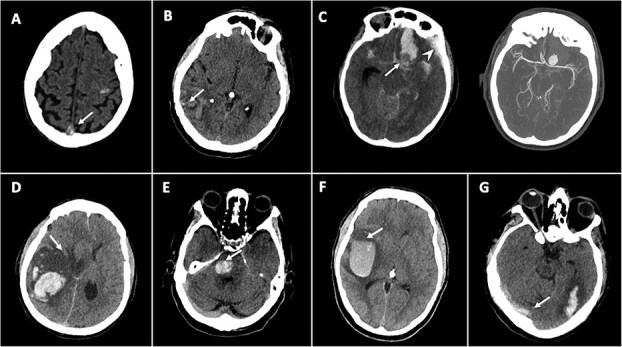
Examples of intra-parenchymal haemorrhagic NCCT findings suggestive of secondary causes of ICH. (A) Small, concave-shaped haemorrhage in the left frontal Rolandic juxtacortical white matter (“cashew nut sign)” with spontaneous hyperdensity of the superior sagittal sinus (arrowhead) suggestive of cerebral venous thrombosis. (B) Right parietal haemorrhage with calcifications (arrow) suggestive of an arteriovenous malformation. (C) NCCT shows a “flame” shape haemorrhage in the left paramedian frontal lobe near a round hypodensity—visible as a concave, “minus” in the haemorrhage (arrow) with associated subdural haemorrhage (arrowhead). Multidetector CTA shows a large saccular aneurysm of the left middle cerebral artery. (D) Large, irregular ICH with a disproportionate perihaematomal oedema (arrow), suggestive of an underlying tumour. (E) Round hyperdense pontine lesion with no surrounding oedema, consistent with cavernoma. (E) Large right temporal haemorrhage with hypodense and hyperdense regions separated by a horizontal line (“fluid-level”) (arrow), consistent with a coagulopathy or haematological disorder. (F) “Flame” shape haemorrhage with spontaneous hyperdensity of right transverse sinus (arrow), suggestive of venous sinus thrombosis. Abbreviation: NCCT = non-contrast CT.
